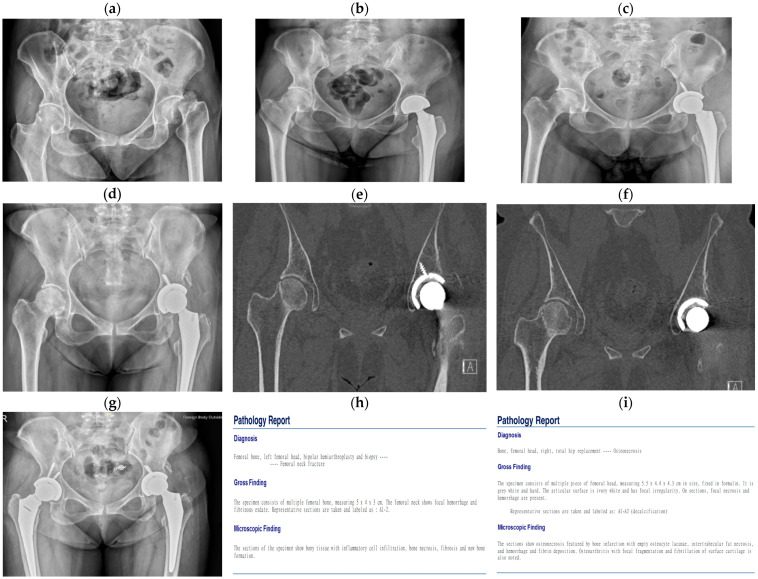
Case 2: A fifty-two-year-old woman with a history of liver cirrhosis and a drinking history of 30 years. (a) Left femoral neck subcapital fracture with displacement. (b) Left hip bipolar hemiarthroplasty for the left femoral neck fracture. (c) Left THA was performed after habitual prosthesis dislocation due to acetabular dysplasia and bone loss at the dislocation site. (d) X-ray showing ONFH over a large area on the right side. (e,f) CT scan in preparation for ONFH staging revealing an impending fracture line in the subcapital area at the same site as the previous left femoral neck fracture. (g) Right THA for right ONFH. (h,i) Pathology reports of bilateral avascular necrosis at both femoral heads.
